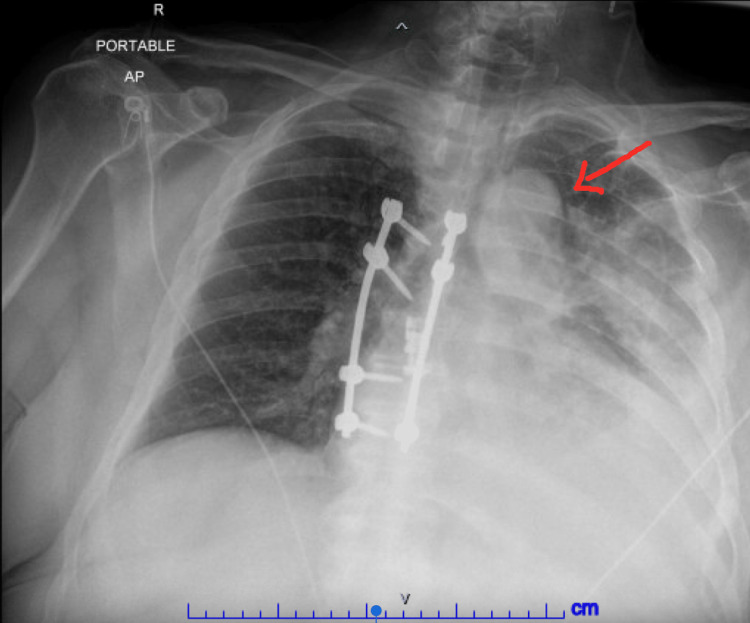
Chest xray revealing left lung mass (Red arrow) with near complete opacification of the left lung
